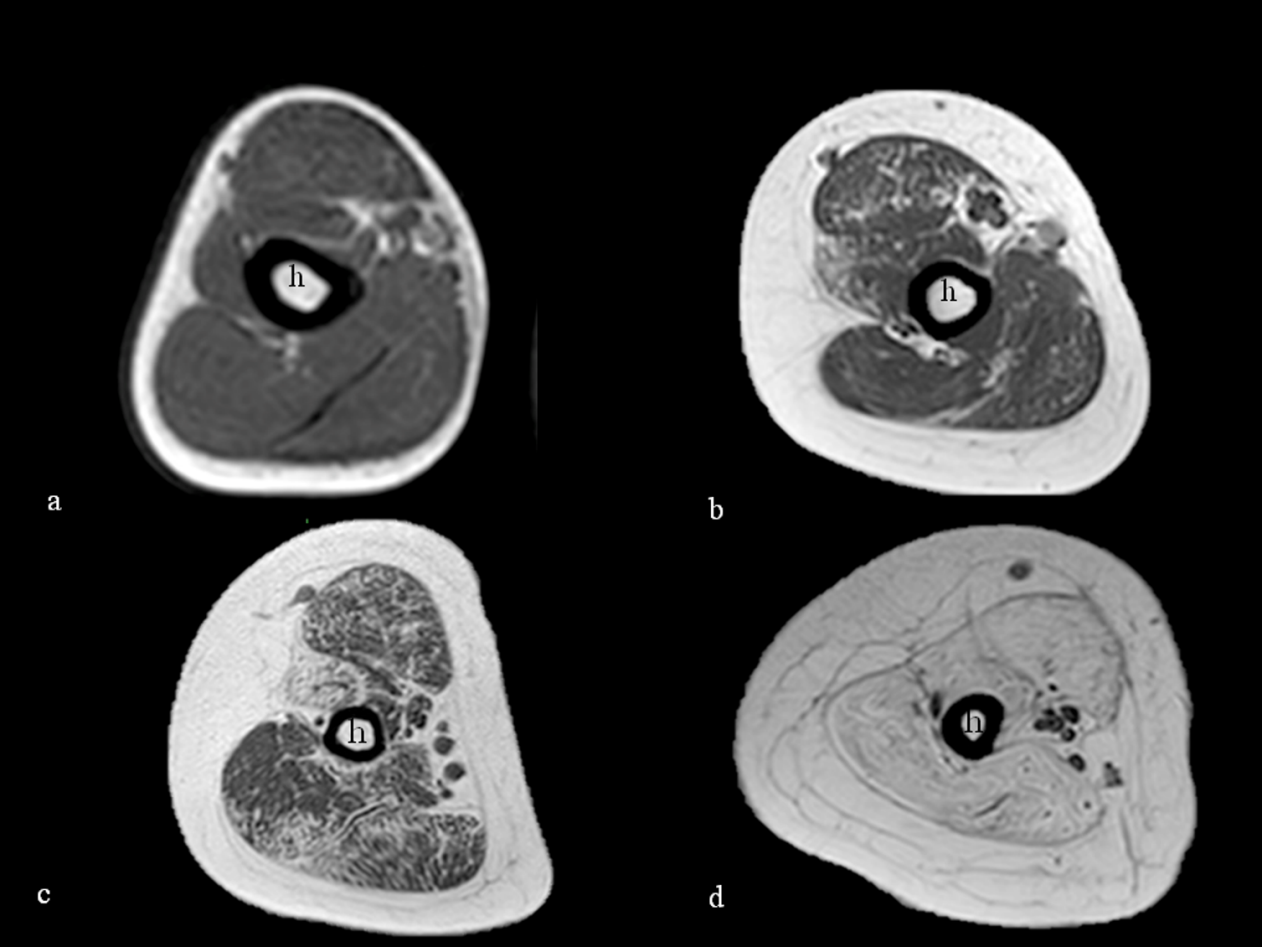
T1-weighted axial MRI of the arm. T1-weighted axial MRI of the arm at the mid-level of the humeral shaft (h) showing progressive increased signal suggestive of fatty replacement in the arm muscles. The involvement is milder in the youngest ambulant patients aged 5 and 11 (a and b respectively) and more marked in the older non-ambulant ones, both 17 years old (c and d) with severe involvement of all the muscles shown in image d.
